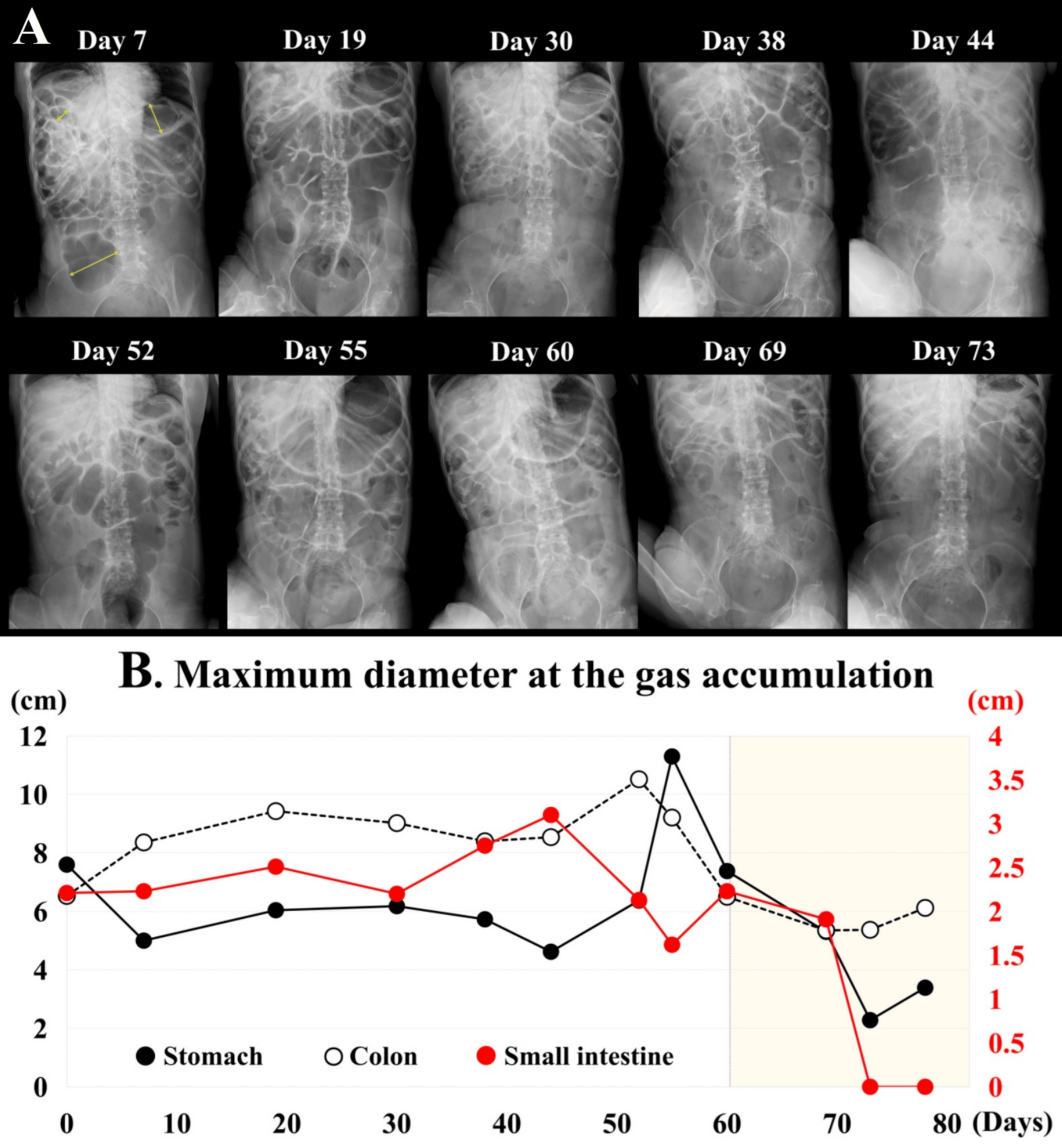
Chronological changes of intestinal gas accumulation (A) Intestinal gas retention on abdominal X-rays Until Day 60, marked gas retention was evident from the stomach to the colon, sometimes resulting in megacolon, with no observed improvement. Once the enteral nutrition conditions were improved through the use of solidifying enteral nutrition and continuous drainage of gastric air via an open nasogastric tube, as described, the gas retention dramatically improved. (B) Measurement of maximum diameters at the gas retention For quantitative analysis, the maximum diameters of the gas accumulation in the stomach, small intestine, and colon were measured using an X-ray system. It is clear that the maximum diameters decreased in the stomach, small intestine, and colon after Day 60, when the procedure was initiated in this case.
